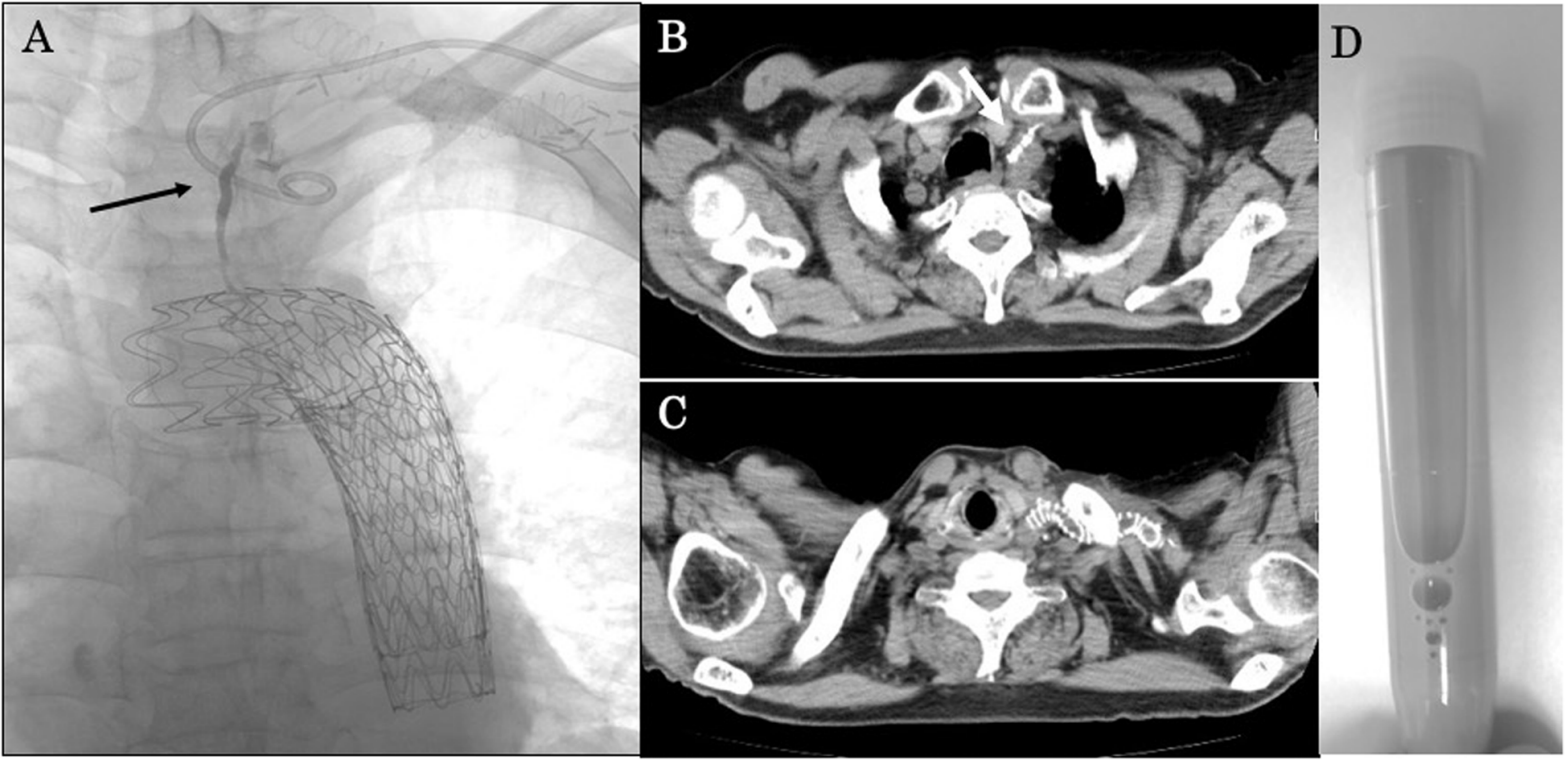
Lymphangiography and computed tomography after treatment. A Lymphangiographic radiography shows a drainage tube, abnormal lipiodol pooling in the chyle leak lumen, and obstruction of the point of leakage of the lymphatic vessel (the arrow). B Computed tomography after treatment revealed obstruction of the point of leakage of the lymphatic vessel (the arrow). C Computed tomography 3 months after discharge shows no reswelling on the neck
